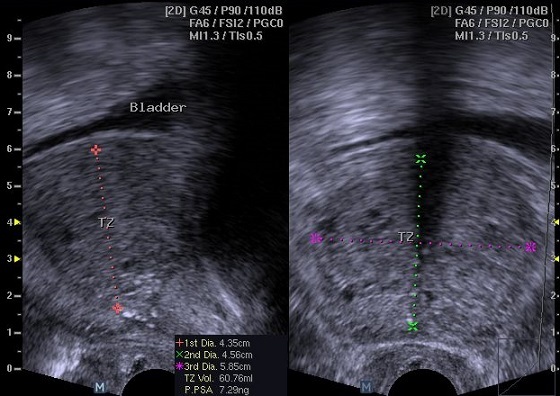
Transrectal ultrasound of the prostate showing measurement of the transitional zone
